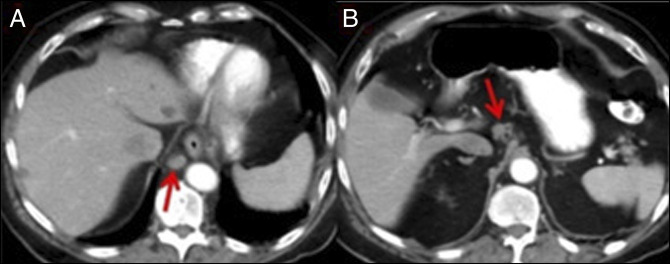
(A) CT showing a newly enlarged right retrocrural lymph node (red arrow) measuring 1.3 cm in the short axis. (B) CT showing newly enlarged celiac axis lymph nodes (red arrow) measuring 1.2 cm in the short axis. CT, computed tomography.
